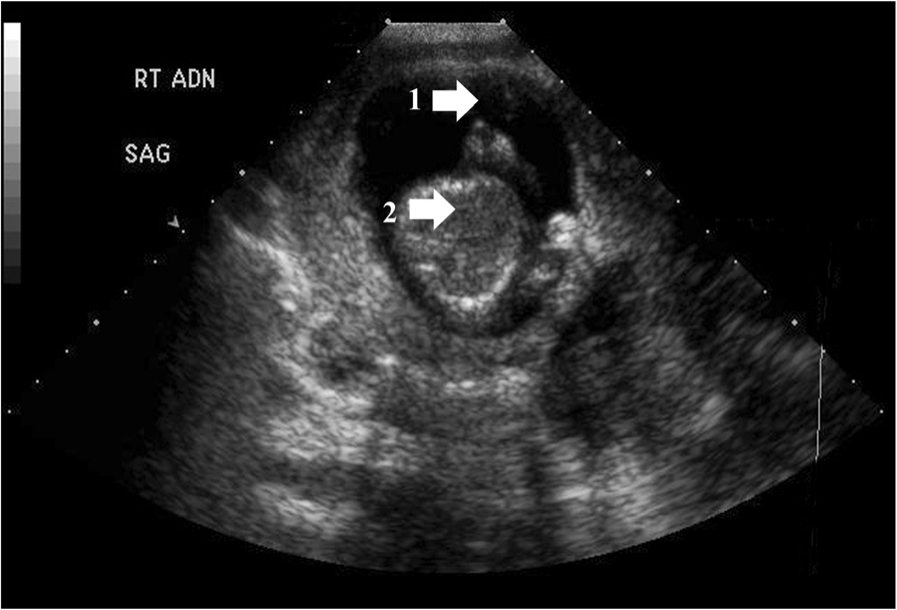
Ultrasound of abdomen and pelvis. 1 Gestational sac. 2 Fetus (extrauterine pregnancy – right adnexa)
